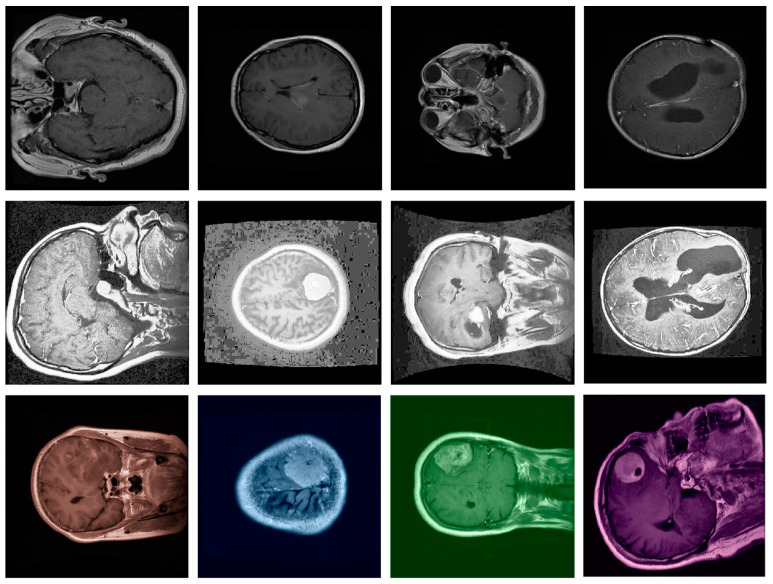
Data preprocessing. The preprocessing steps applied to the MRI images in our study. These steps include normalization, resizing, histogram equalization, and data augmentation, which are essential for standardizing image quality and enhancing contrast between tumor and non-tumor regions. By ensuring consistent input, these preprocessing techniques improve the robustness of the model, allowing it to perform more accurately across diverse MRI scans.
